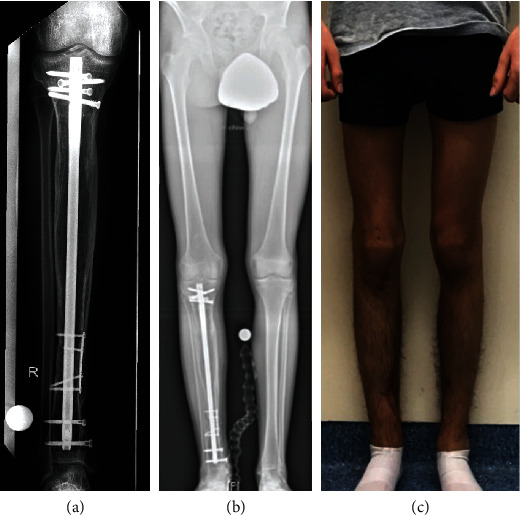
Patient 2. (a) Radiograph showing complete consolidation of the entire tibia including the former callotasis site and the distal docking site with autologous bone grafting harvested from the iliac crest as well as trauma nail and locking plate osteosynthesis. (b) Bilateral long-standing radiograph showing correct alignment and length of the reconstructed leg. (c) Clinical presentation showing equal leg lengths.
